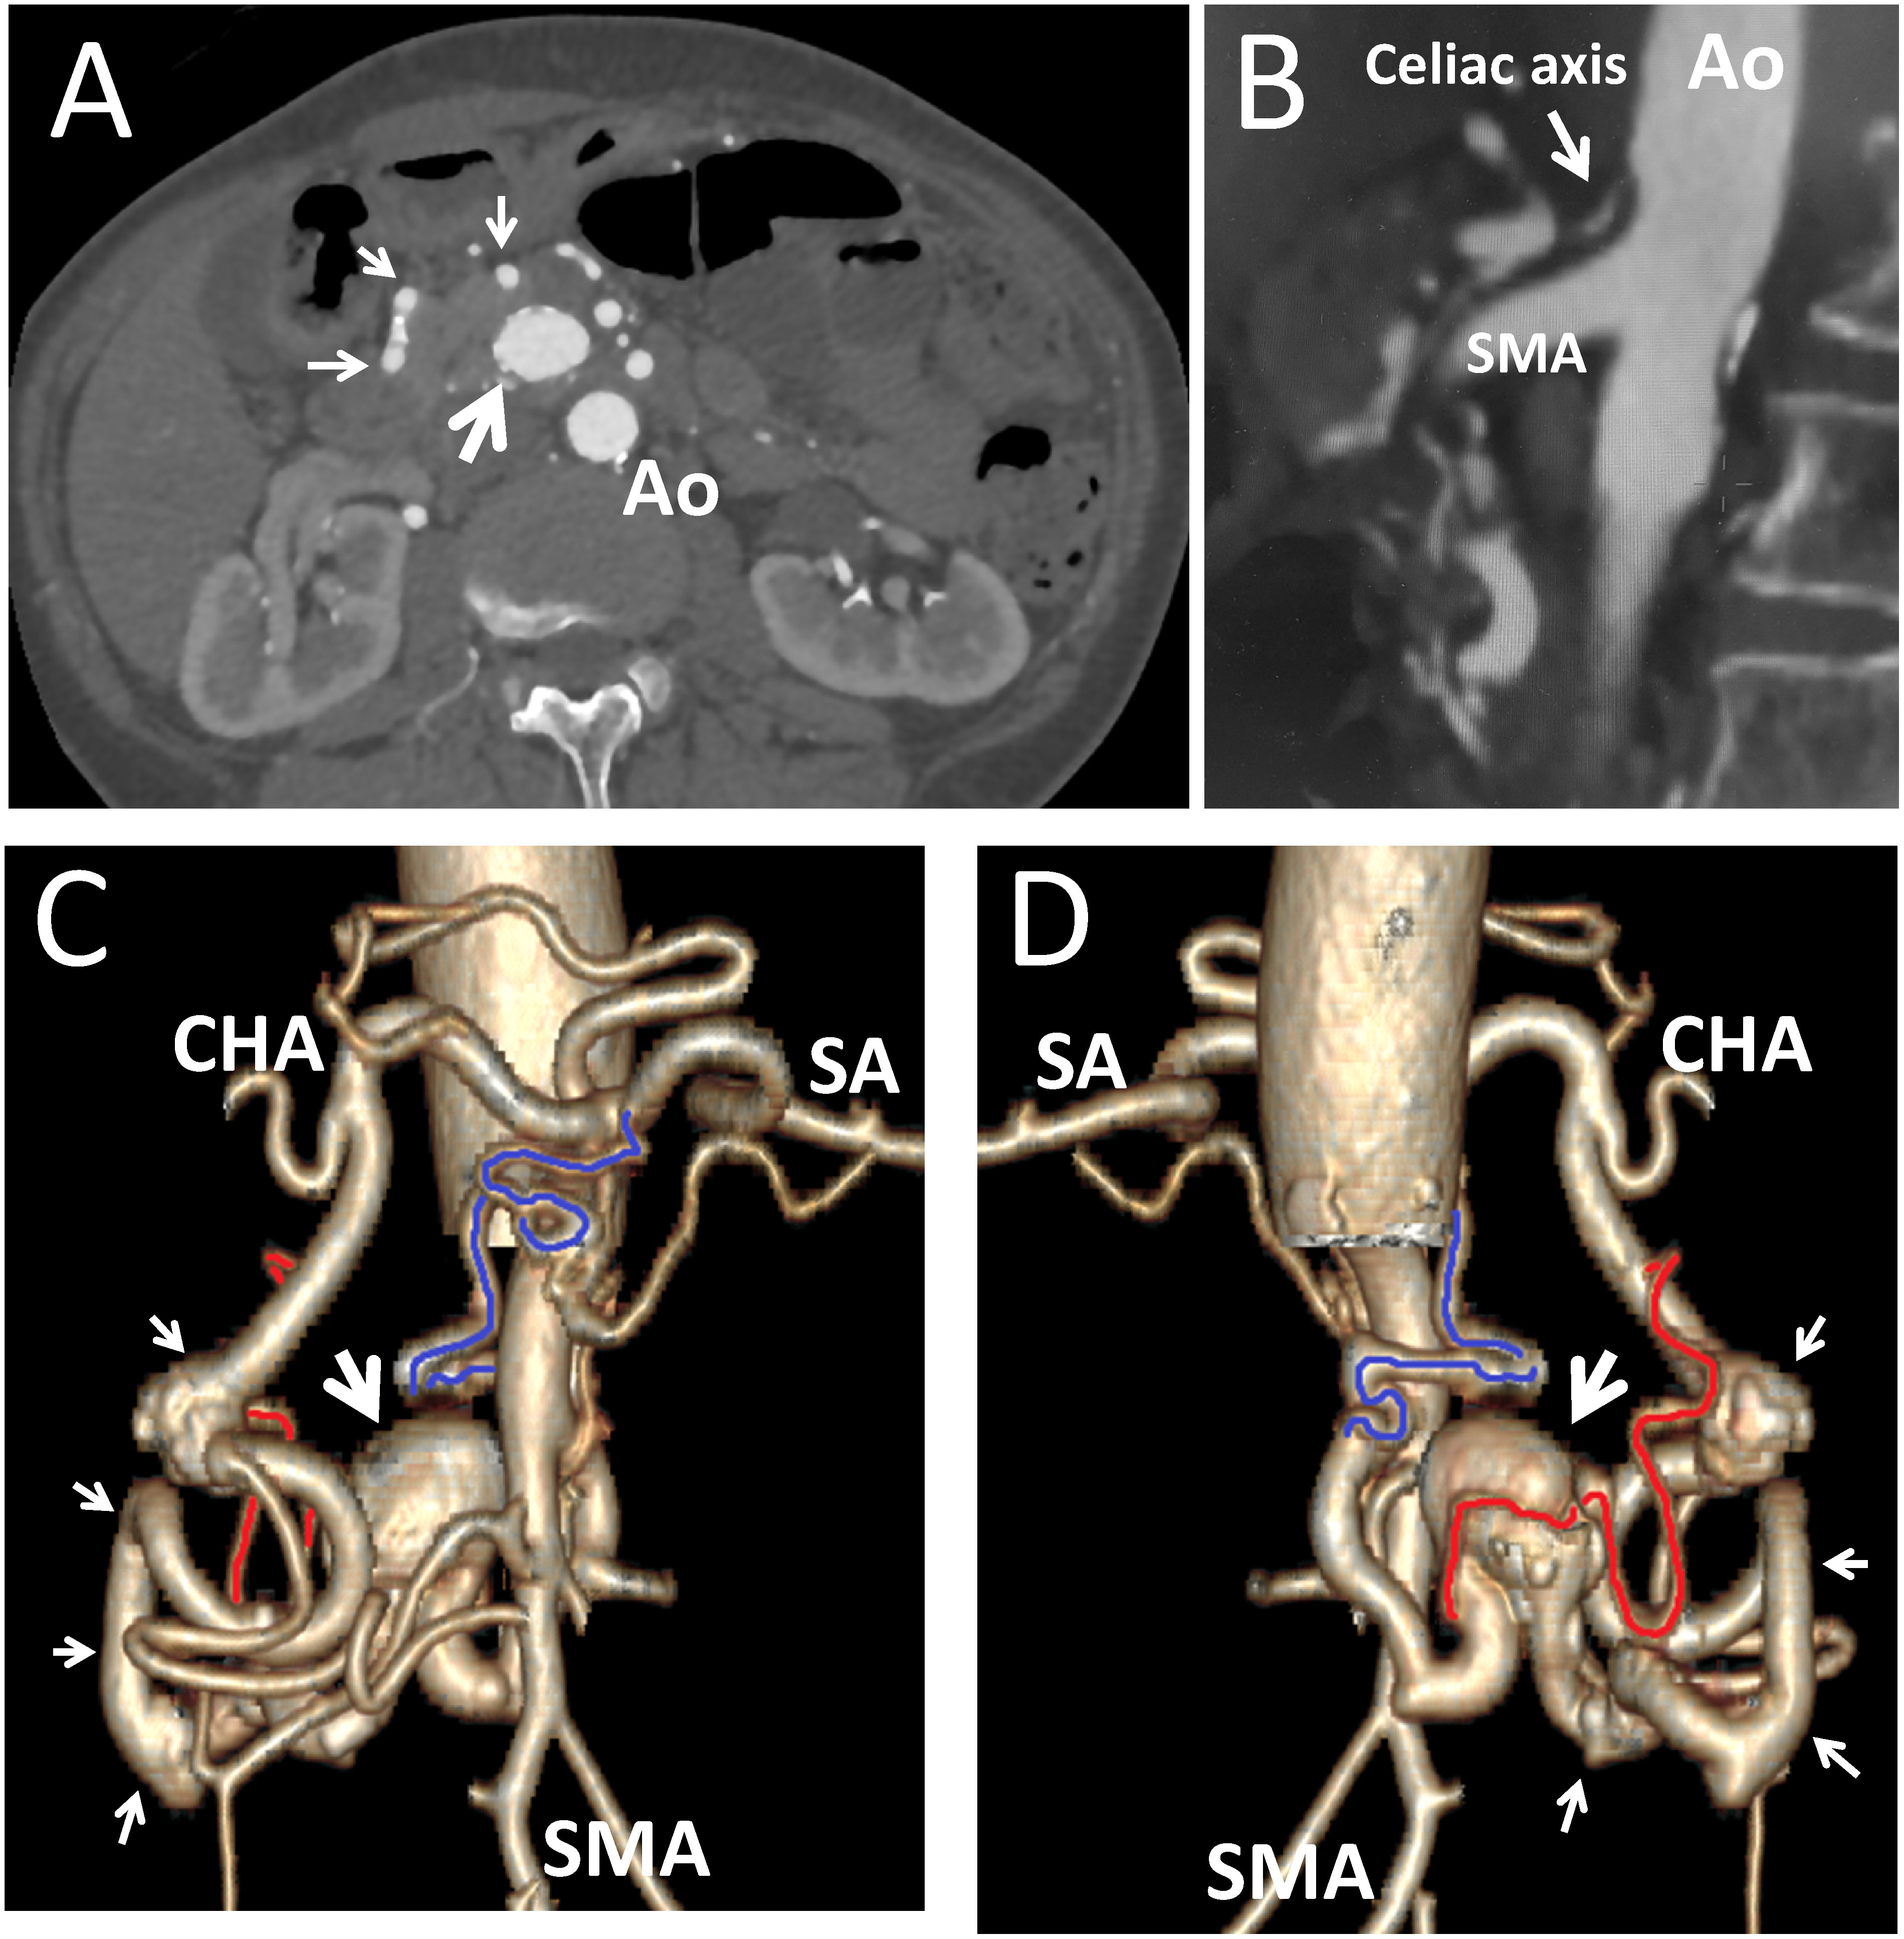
Fig. 2 Preoperative computed tomography (CT) images for patient 2. (A) Preoperative CT slice demonstrating the pancreaticoduodenal artery aneurysm (large arrow). The dilated anterior superior pancreaticoduodenal artery (ASPDA) is also visible (small arrows). (B) The axis of the celiac artery was occluded. (C, D) View from the front (C) and the back (D) in the preoperative three-dimensional CT images. In addition to the ASPDA (small arrows), the posterior superior pancreaticoduodenal artery (red lines) and the dorsal pancreatic artery (blue lines) are visible as collateral pathways connecting the SMA and the celiac artery. Large arrow: the aneurysm.
